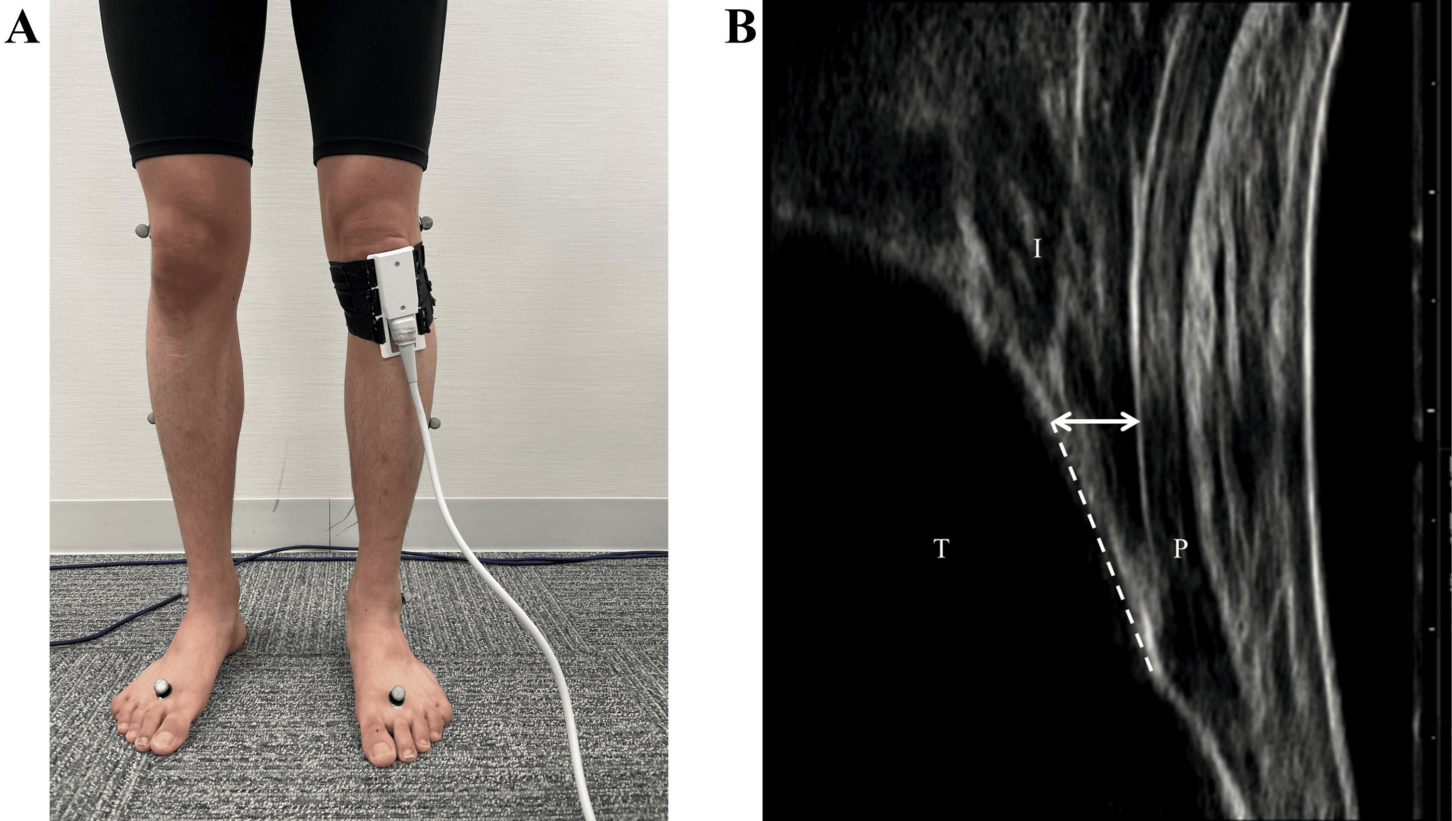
Transducer and ultrasound images (A) Snapshot of a transducer fitted to the anterior surface of the knee; (B) ultrasound image of the infrapatellar fat pad. I: infrapatellar fat pad; T: tibia; P: patellar tendon; dashed line: 10 mm line on a tibial cortex from the tendon insertion; arrow, IFP thickness.
